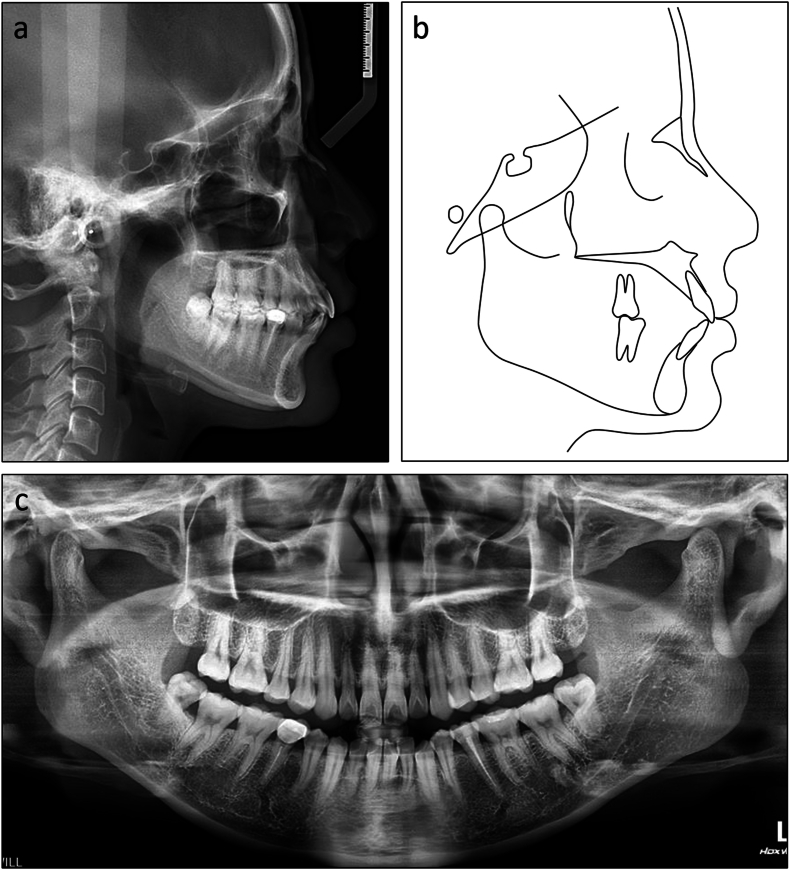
Pretreatment radiographs and cephalometric tracing of Case 1. (a) Cephalometric radiograph. (b) Cephalometric tracing. (c) Panoramic radiograph.
